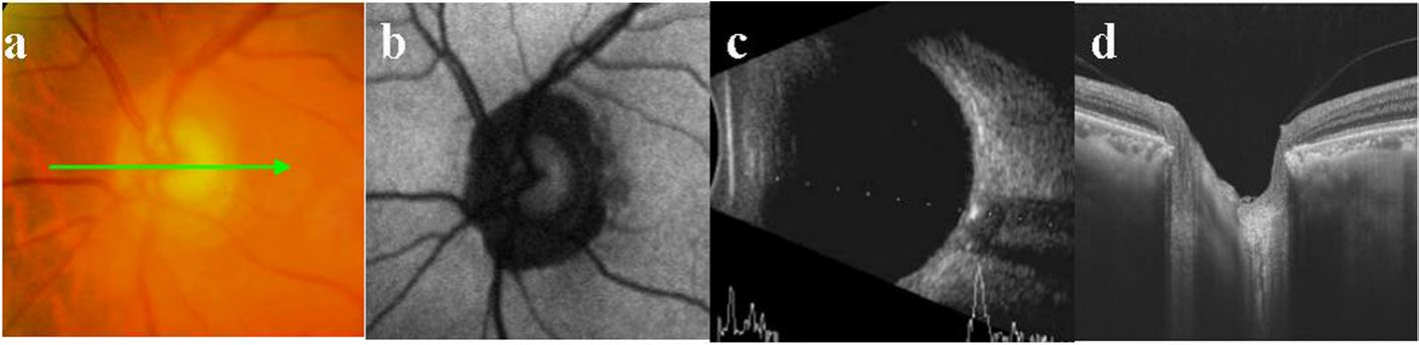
Multimodal imaging of a special buried ODD ODD was confirmed by B-ultrasound, however, no special finding was noticed in the fundus photography, no noticeable autofluorescence and abnormality was seen in SS-OCT
